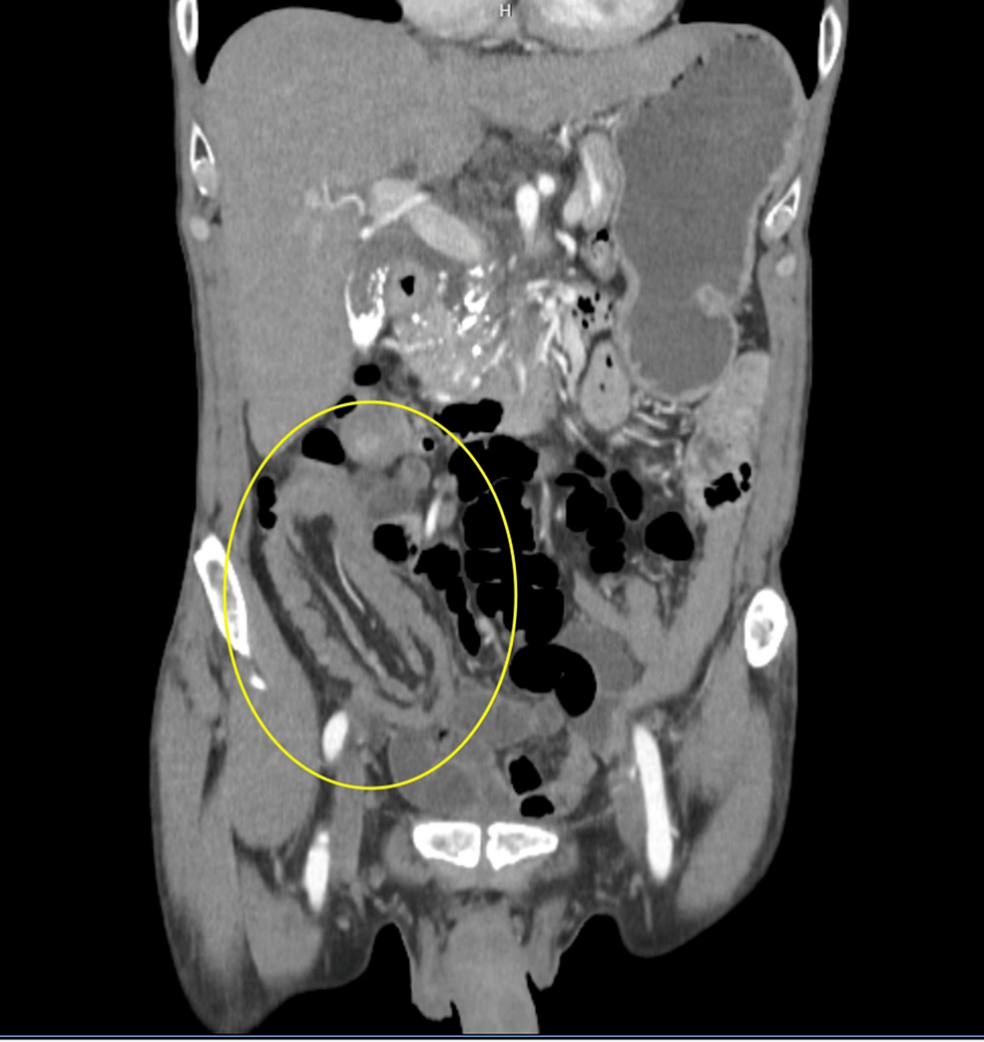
Ileocecal intussusception with cecal soft tissue thickening found on CT imaging (Yellow circle)
